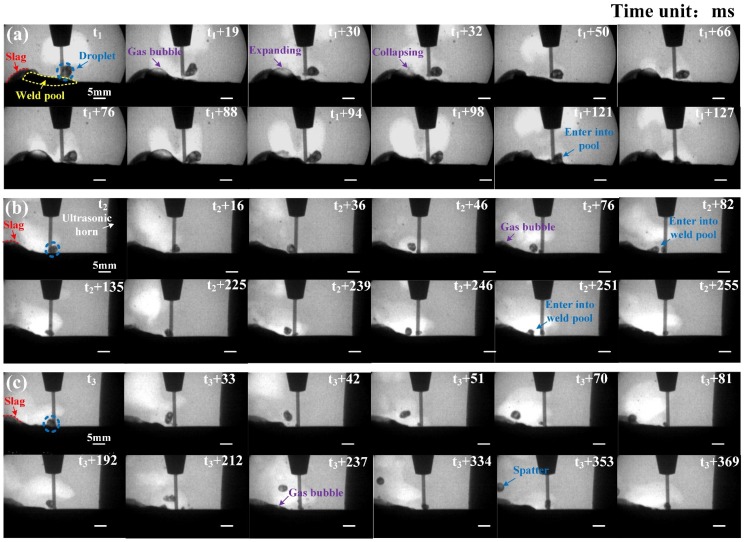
X-ray images of melt flow and droplet transfer process obtained at different conditions: (a) without ultrasonic; (b,c) ultrasonic output power was 40% and 80% of the maximum power (1200 W), respectively.
